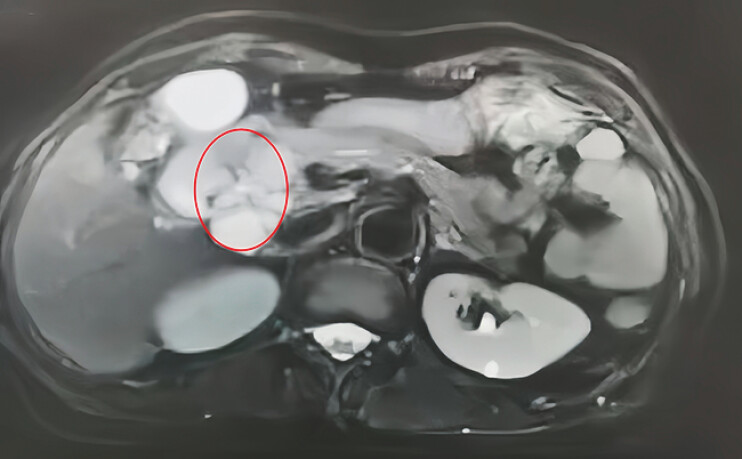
A MRI scan revealed a mass in the pancreatic head, suggesting a serous cystadenoma. MRI, magnetic resonance imaging.
